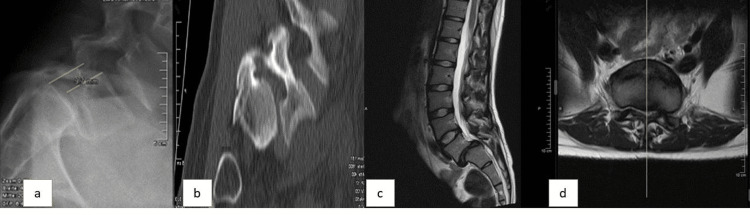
(a) Lateral X-ray with III° spondylolisthesis. (b) Sagittal CT view showing the pars elongation and the dysplastic pedicle. T2 MRI showing the foraminal (c) and central (d) stenosis.
